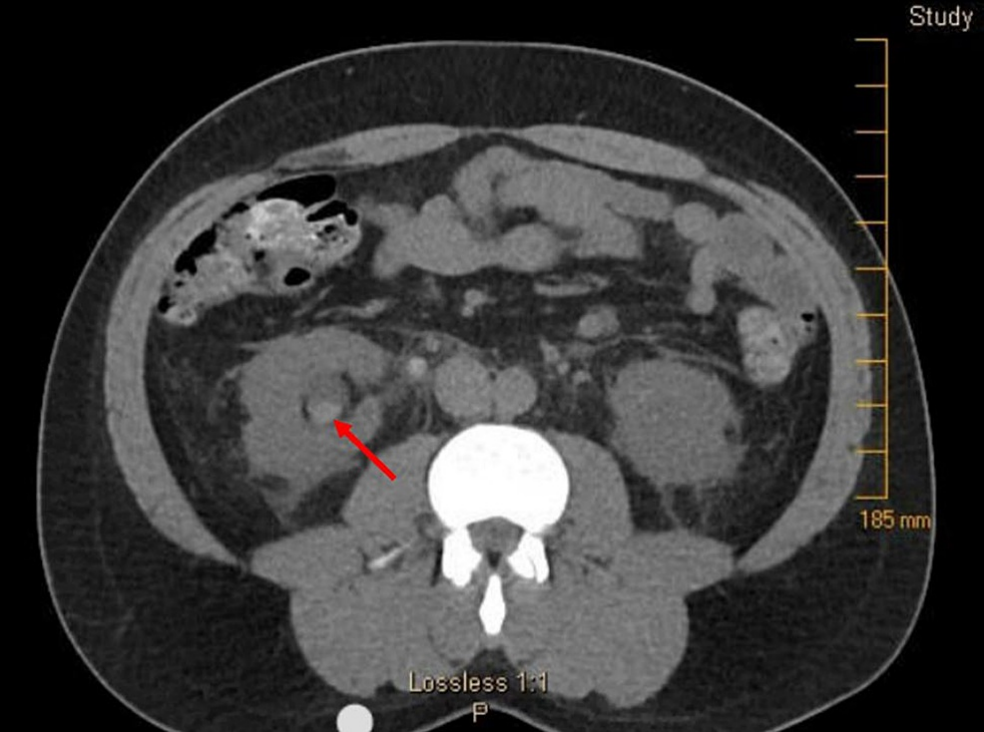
Abdominal and pelvic non-contrast CT revealing hyperdense material in right lower pole renal calyx.
